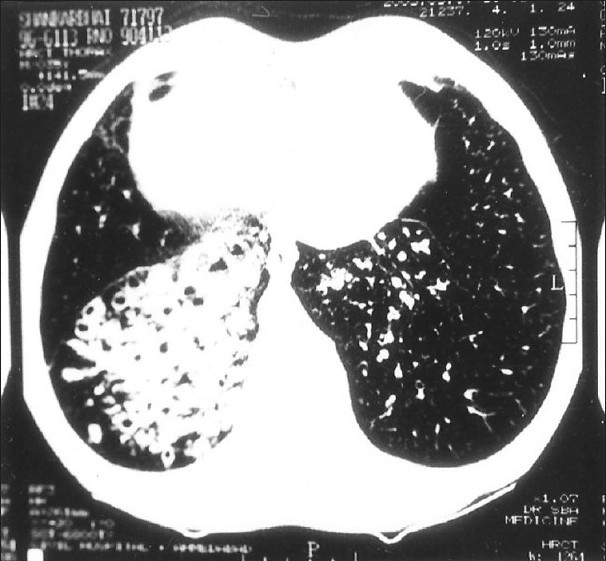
CT scan chest showing bronchiectatic changes and consolidation at lower lobes (more on right side)
